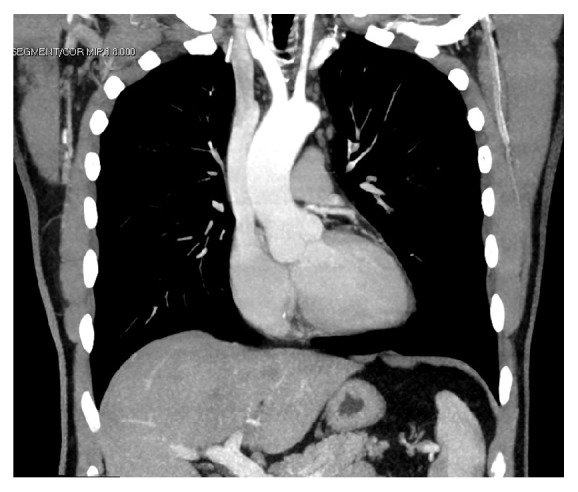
Postoperative contrast CT scan of the thorax demonstrating normal appearance of the right and left sinuses of Valsalva.
